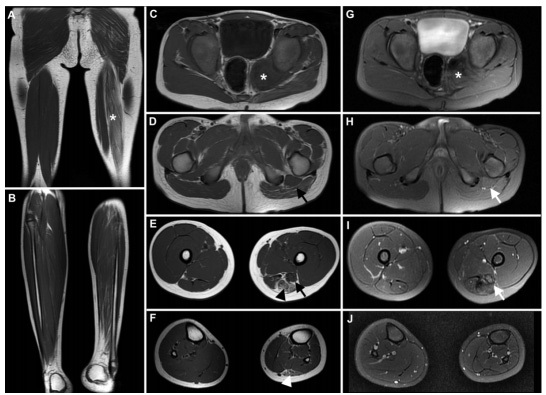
Muscle magnetic resonance (MR) imaging studies of the lower limbs. Coronal T1-weighted MR image of the thighs shows fatty substitution of the long head of the biceps femoris on the left thigh (asterisk) (A). Coronal T1-weighted MR image of the lower legs shows substantial asymmetry with diffuse hypotrophy of the left lower leg muscles in comparison to the contralateral unaffected side (B). Axial T1-weighted images of the pelvis (C), thighs (D and E) and lower legs (F) show a mass in the pelvis (C; asterisk) that merges with the left sciatic nerve (D and E; black arrows), which shows fusiform enlargement, with homogeneous intermediate signal intensity, similar to the adjacent muscle bellies. The fatty replacement of the long head of the biceps femoris (E; black arrowhead) and of the lateral head of the left gastrocnemius (F; white arrowhead) are shown. Axial T2-weighted fat-saturated images show intermediate signal intensity of the pelvic mass (G; asterisk) and the enlarged sciatic nerve (H and I; white arrows). Homogeneous Intermediate signal in T2- and T1-weighted images similar to adjacent muscle bellies is highly suggestive of neuromuscular choristoma (asterisk and arrows).
